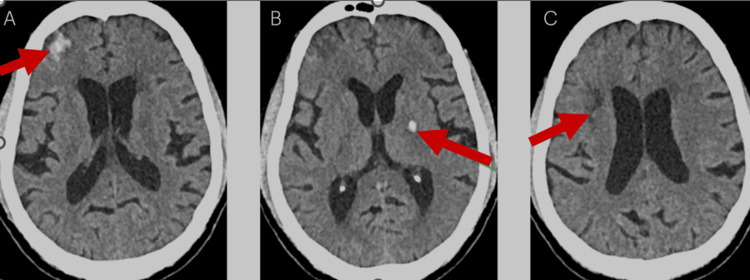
CT of the brain (A) The red arrow highlights the intraparenchymal hemorrhage in the right basifrontal lobe with surrounding edema. (B) The red arrow highlights the hemorrhagic focus in the posterior limb of the left internal capsule. (C) The red arrow highlights a subacute to chronic nonhemorrhagic infarct in the right corona radiata. CT: computed tomography.
